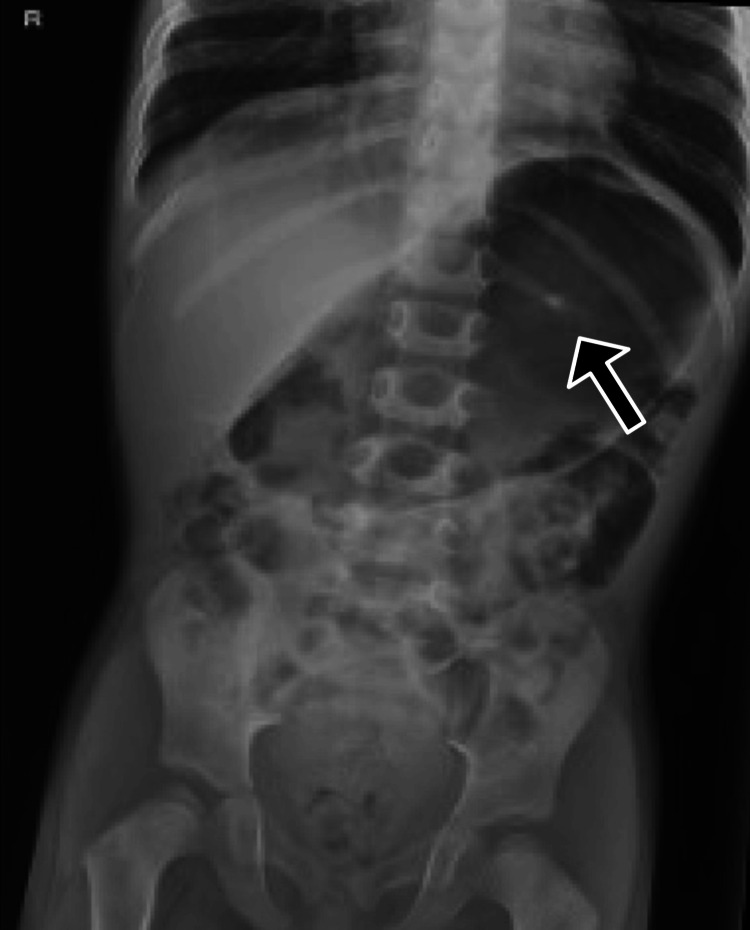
KUB radiograph with an arrow indicating the ingested tooth visualized in the stomach KUB: kidneys, ureters, and bladder
